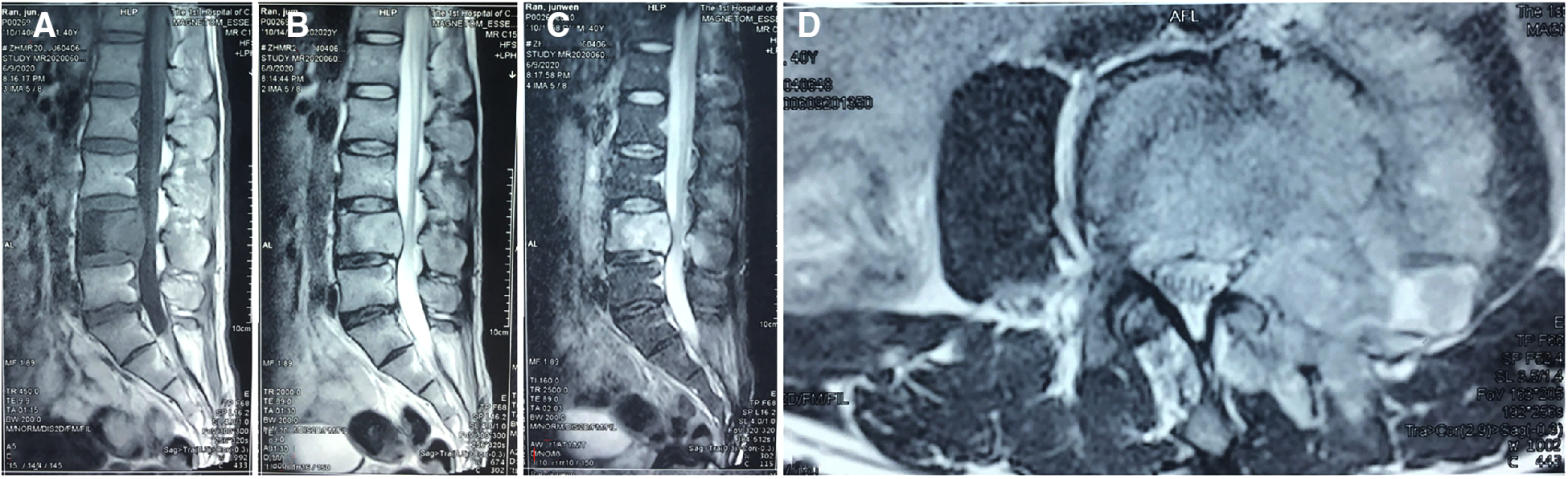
MRI scan showing the paravertebral soft tissue mass and the spinal canal stenosis and the pedicle of the fourth lumbar vertebra. (A) T1-weighted images, (B) T2-weighted images, (C) short tau inversion recovery, and (D) transverse section imaging.
